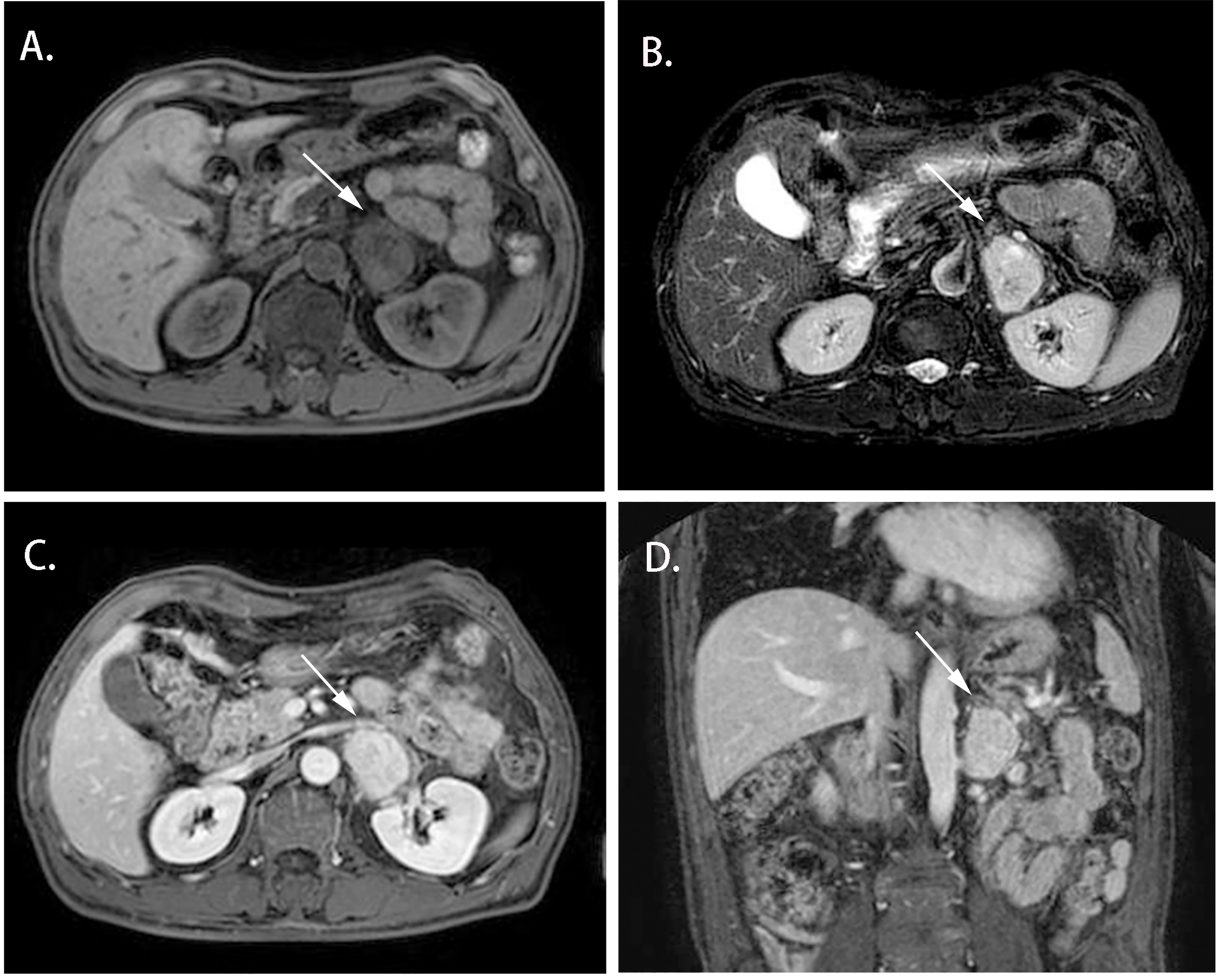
Magnetic resonance imaging of the Adrenal CD of Case 2 (white arrow). (A) Axial T1WI; (B) Axial T2WI; (C) Enhanced T1W1; (D) Coronal Enhanced T1W1. MRI scan and dynamic enhancement showed a 46× 33× 40 mm mass with hypo-intensity signal on T1WI and hyper-intensity on T2WI and with mild to moderate heterogeneous enhancement in the left adrenal region.
